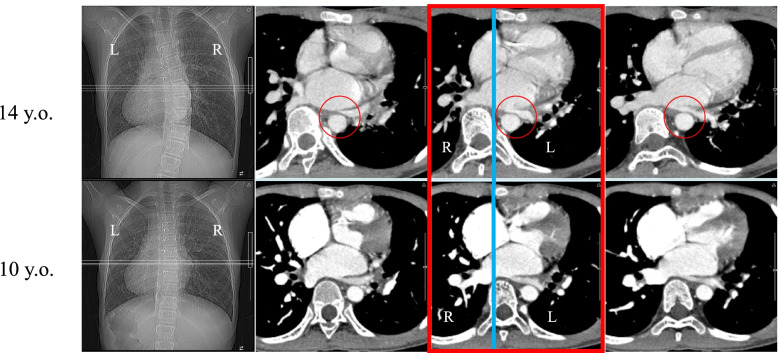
Preoperative contrast CT. Lateral deviation and right anterior rotation of the T8 vertebral body were evident. The descending aorta appeared to be draining in close proximity to the left pulmonary vein (circles)
